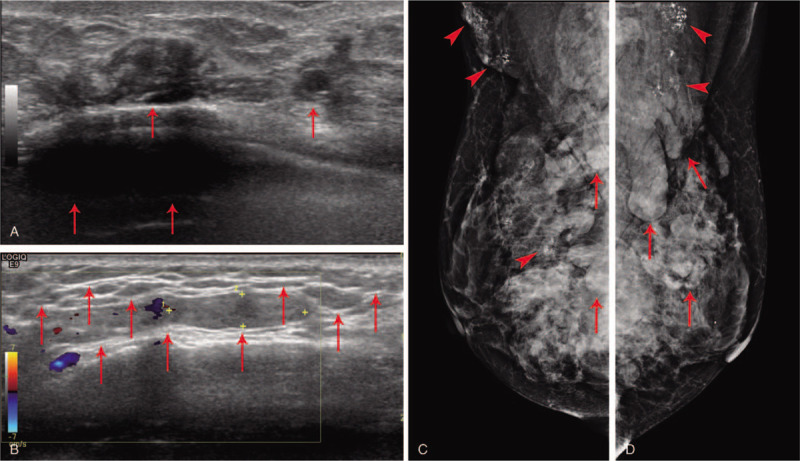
(A, B) Ultrasonography. Multiple hypoechoic lesions suggestive of cystic masses and diffuse infiltrations were observed (arrow). (C, D) Mammography. Multiple, irregularly-shaped masses (arrow) and calcification (arrow head) were observed in both breasts and axillas.
